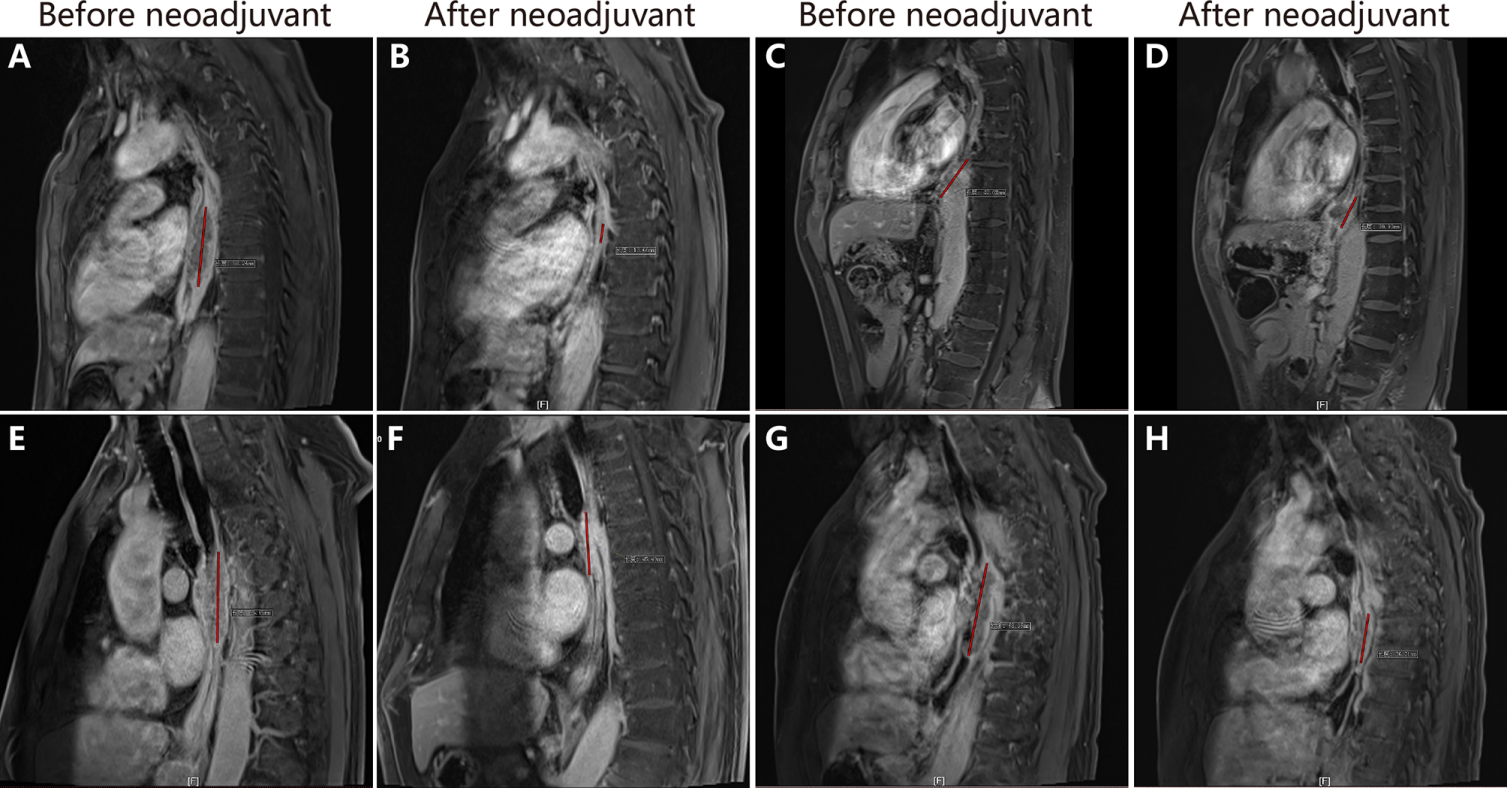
The changes of esophageal lesions in magnetic resonance imaging. (A, B) show the esophageal lesion in patient 4 before and after neoadjuvant chemoimmunotherapy, the lesion reduced significantly. (C, D) show esophageal lesion in patient 7 before and after neoadjuvant chemoimmunotherapy. (E, F) show esophageal lesion in patient 1 before and after neoadjuvant chemoimmunotherapy. (G, H) show esophageal lesion in patient 8 before and after neoadjuvant chem.
